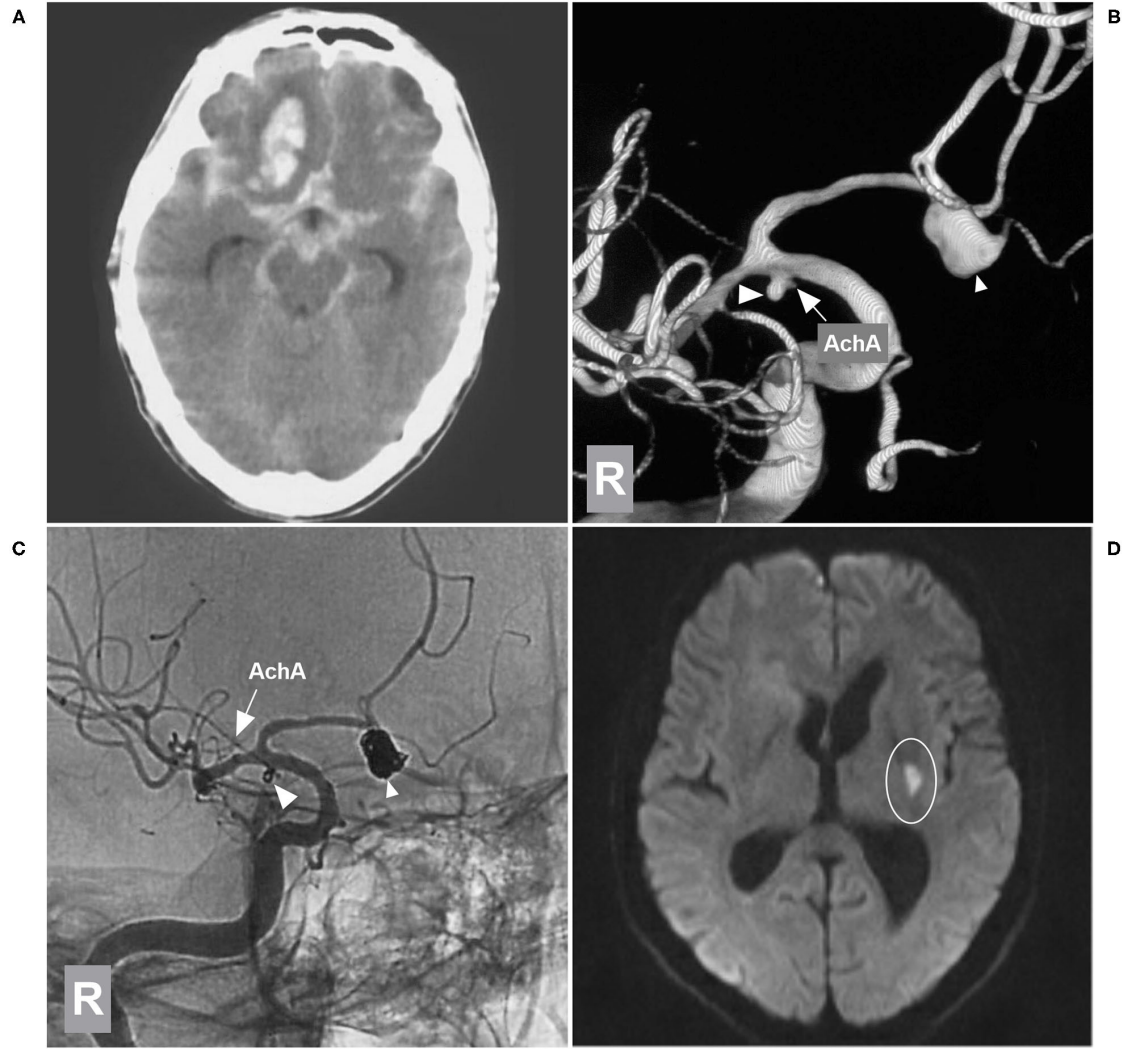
EVT of the AchA aneurysm with brain infarction. (A) CT showing subarachnoid hemorrhage with a hematoma in the right frontal lobe base. (B) 3D-DSA of the right ICA showing type II AchA aneurysm (large triangle) and AcomA aneurysm (small triangle), and the arrow indicates the AchA. (C) Unsubtracted DSA of the right ICA showing that two aneurysms were coiled (large and small triangles); the AchA (arrow) was intact. (D) MRI DWI sequence showing the acute infarction (circle region) in the posterior limb of the internal capsule. AchA, anterior choroidal artery; AcomA, anterior communicating artery; CT, computed tomography; DSA, digital subtraction angiography; DWI, diffusion-weighted imaging; EVT, endovascular treatment; ICA, internal carotid artery; MRI, magnetic resonance imaging; R, right; 3D, three-dimensional.
